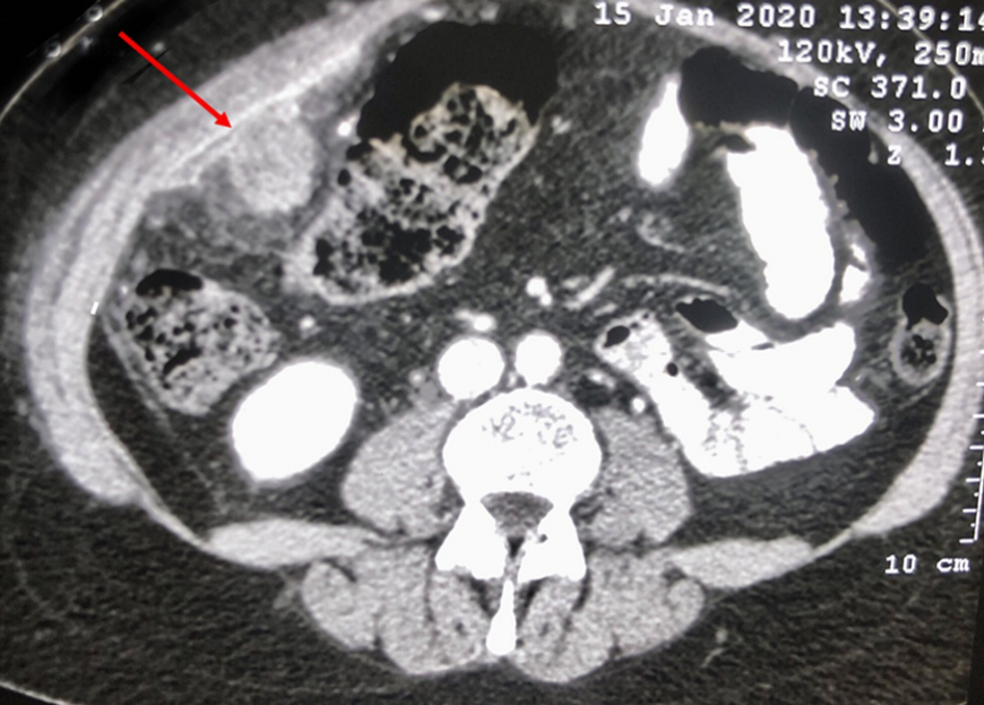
Computed tomography scans with contrast enhancement demonstrating the ill-defined enhanced soft tissue tumour noted posterior to the right anterior abdominal wall (red arrow indicates tumour).
